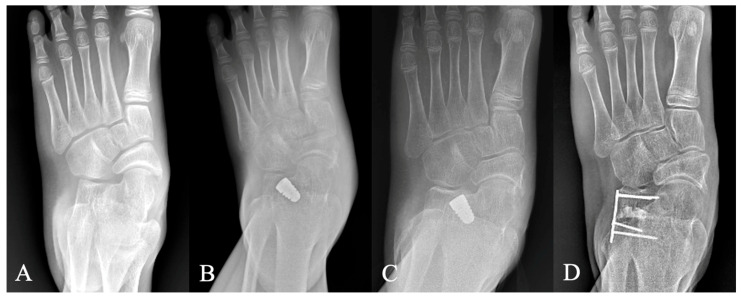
Implant subsidence in a 10-year-old girl who underwent STA and later sustained an ankle sprain. (A) A preoperative AP radiograph of the left foot showing forefoot abduction deformity. (B) An AP radiograph at 7 months postoperatively reveals subsidence of the STA implant following a sprain injury. (C) A radiograph taken 1 year postoperatively also demonstrates implant subsidence. (D) Bone union was achieved following revision surgery with lateral column lengthening.
